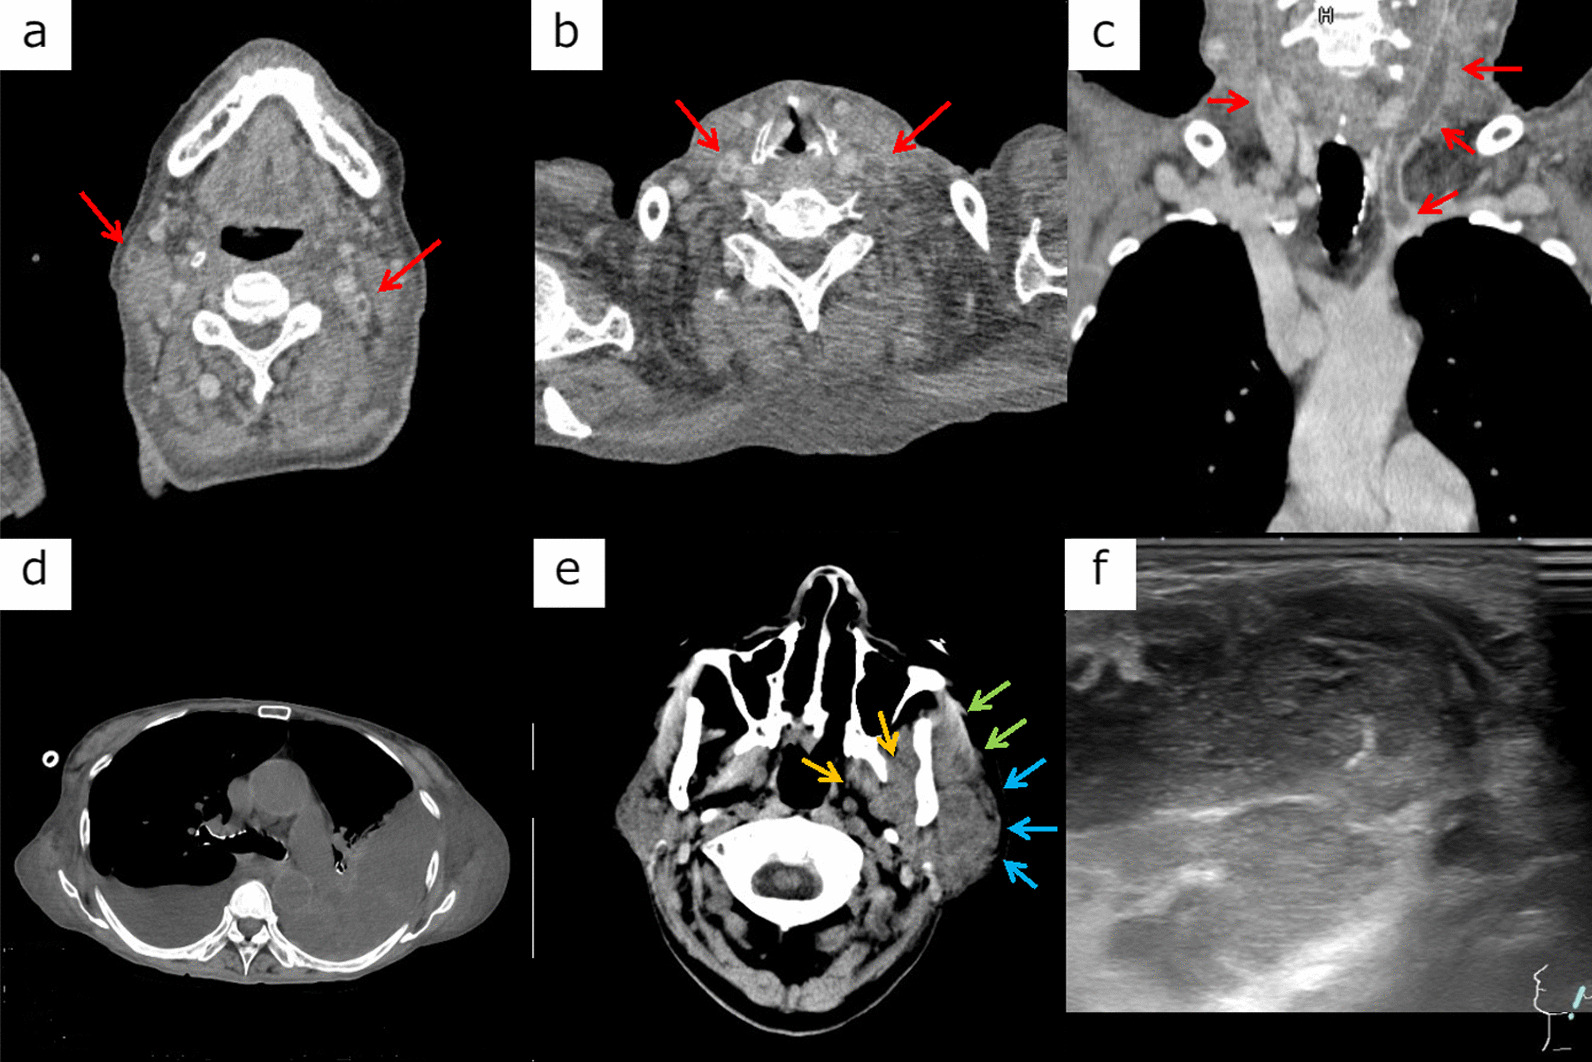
a–c On the 8th day, thrombi appeared in the right external jugular vein, bilateral internal jugular veins, and the right small saphenous vein. d, e On the 10th day, a left hemothorax appeared, and the left parotid gland (blue arrow), near the pterygoid muscle group (yellow arrow) and masseter muscle (green arrow), was enlarged. A slightly low internal absorption, suggesting abscess formation, was also observed. f Posterior echogenic enhancement with a well-defined border and heterogeneous internal echogenicity of about 37 × 35 mm near the left parotid gland, with findings showing an abscess after superficial ultrasound examination of the left parotid gland
